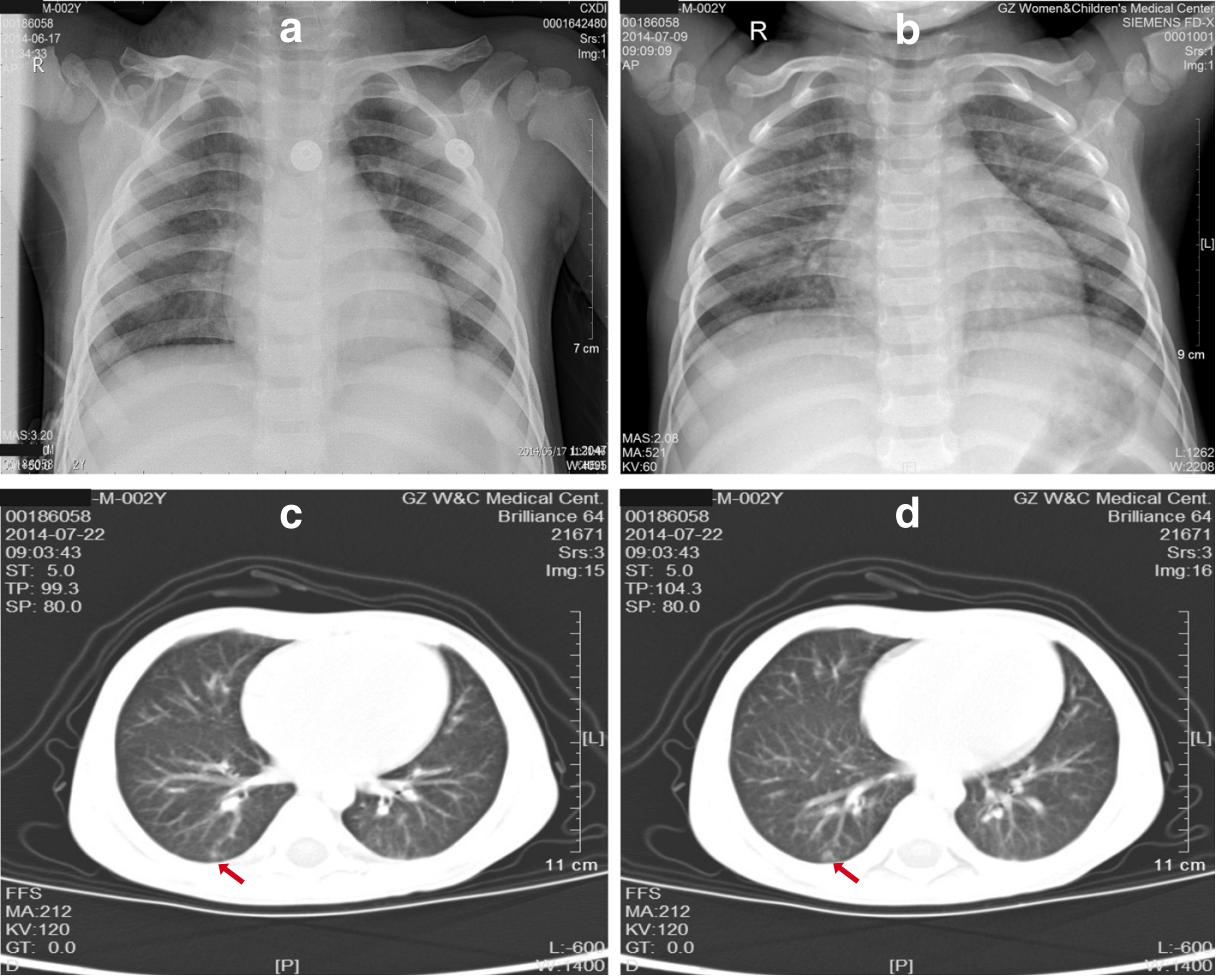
The results of chest X-ray, CT of patient. The chest X-ray images showed slightly increased lung-markings from Jun 17th to July 9th (Panels a, b), and images of chest CT revealed pneumonia in the posterior basal segment oflower lobe of right lung on July 22nd (Panels c, d)
